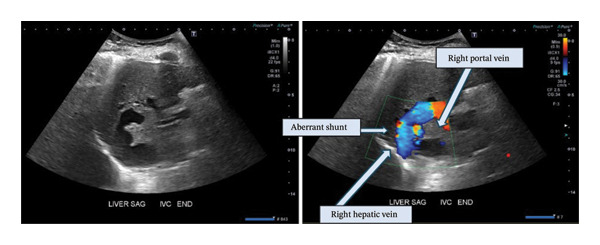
Representative ultrasound images of dominant right portal‐hepatic veno‐venous shunt in greyscale and color doppler.
